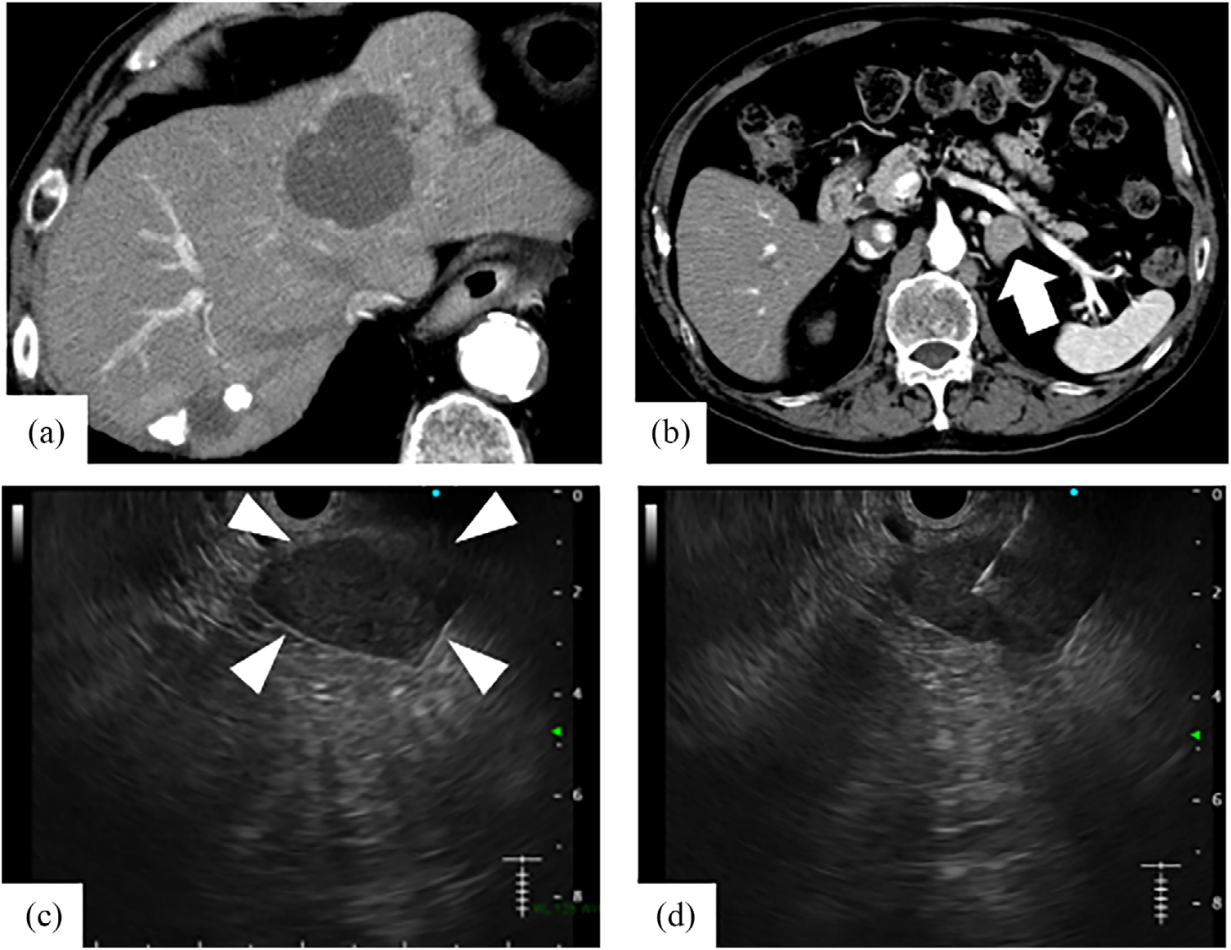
(a, b) Computed tomography showed a tumor with 23 mm in diameter at the left adrenal gland, suggesting the possibility of an adrenal metastasis from lung adenocarcinoma, hepatocellular carcinoma, or a primary adrenal gland tumor (white arrow). And liver tumors were not detected in liver segments 4 and 7 on computed tomography. (c, d) Endoscopic ultrasound shows a hypoechoic mass in the left adrenal gland (white arrowheads). We performed endoscopic ultrasound‐guided fine‐needle aspiration of the left adrenal gland using a 25‐gauge needle.
